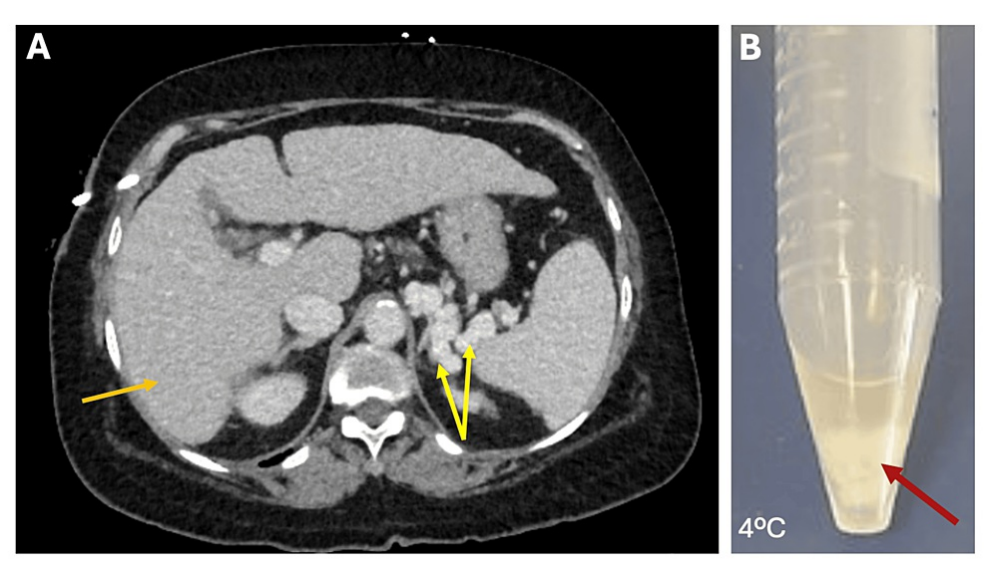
Representative images from abdominal CT and serum sample showing a characteristic cryocrit formation. A. Abdominal CT showed the presence of the liver with cirrhotic morphology (orange arrow), as well as with multiple left upper quadrant varices consistent with portal hypertension (yellow arrows). B. Serum sample showing a characteristic cryoglobulin pellet present at 4°C (red arrows).
